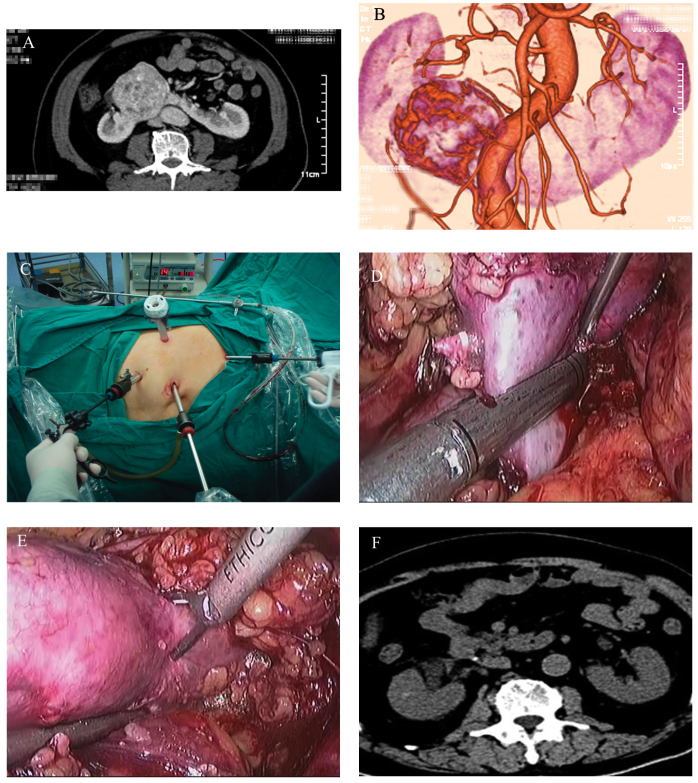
(A and B) Pre-operative computed tomography angiography (CTA). (C) The location of the port sites during surgery. (D) The ismthus was divided using the endoscopic stapler. (E) The tumor was excised with ultrasonic scissors. (F) CTA demonstrating no signs of disease relapse subsequent to an 18-month follow-up.
